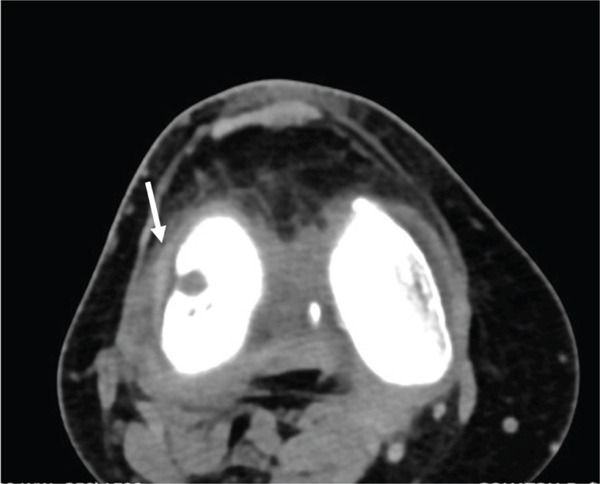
Preoperative computed tomography views of the right knee further confirm the osteochondral defect at the lateral aspect of the lateral femoral condyle (white arrow), measuring approximately 1.4 × 1.2 × 0.5 cm.
